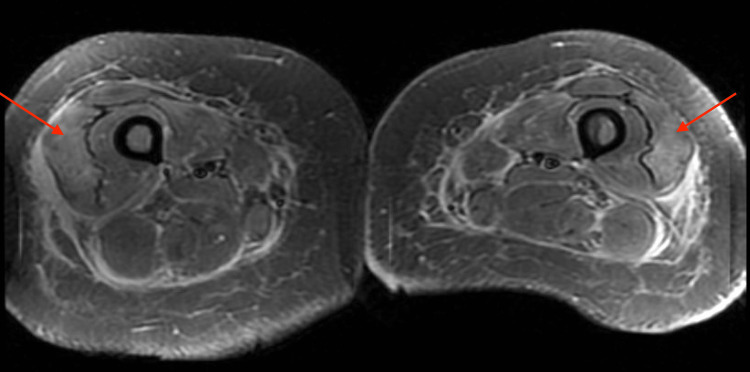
MRI of the lower extremities showing bilateral patchy hyperintense signal in the lateral compartment. Moderate myositis.
